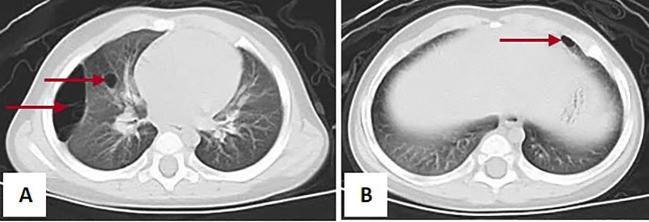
Images of the chest CT scan showed lesions in both lungs. (A) Right lung. (B) Left lung. The red arrow in Panel (A) points to the lesion in the right lung, and the red arrow in Panel (B) points to the lesion in the left lung.
